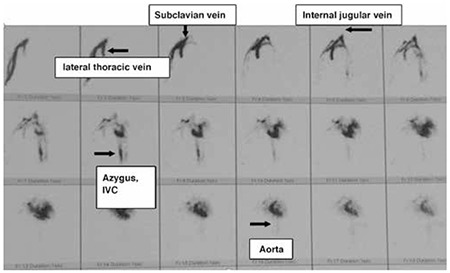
Angioscintigraphy anterior view of the chest after bolus injection of 5 mCi 99mTechnetium-diethylenetriaminepentaacetic acid through the same venous cannula. Sequential 1-second images showed rapid filling of the right basilic and cephalic veins, followed by axillary and subclavian veins. Two parallel channels appeared and then the right heart, left heart and aorta became visible in turn. Regurgitation into the lateral thoracic and internal jugular veins was also noted
